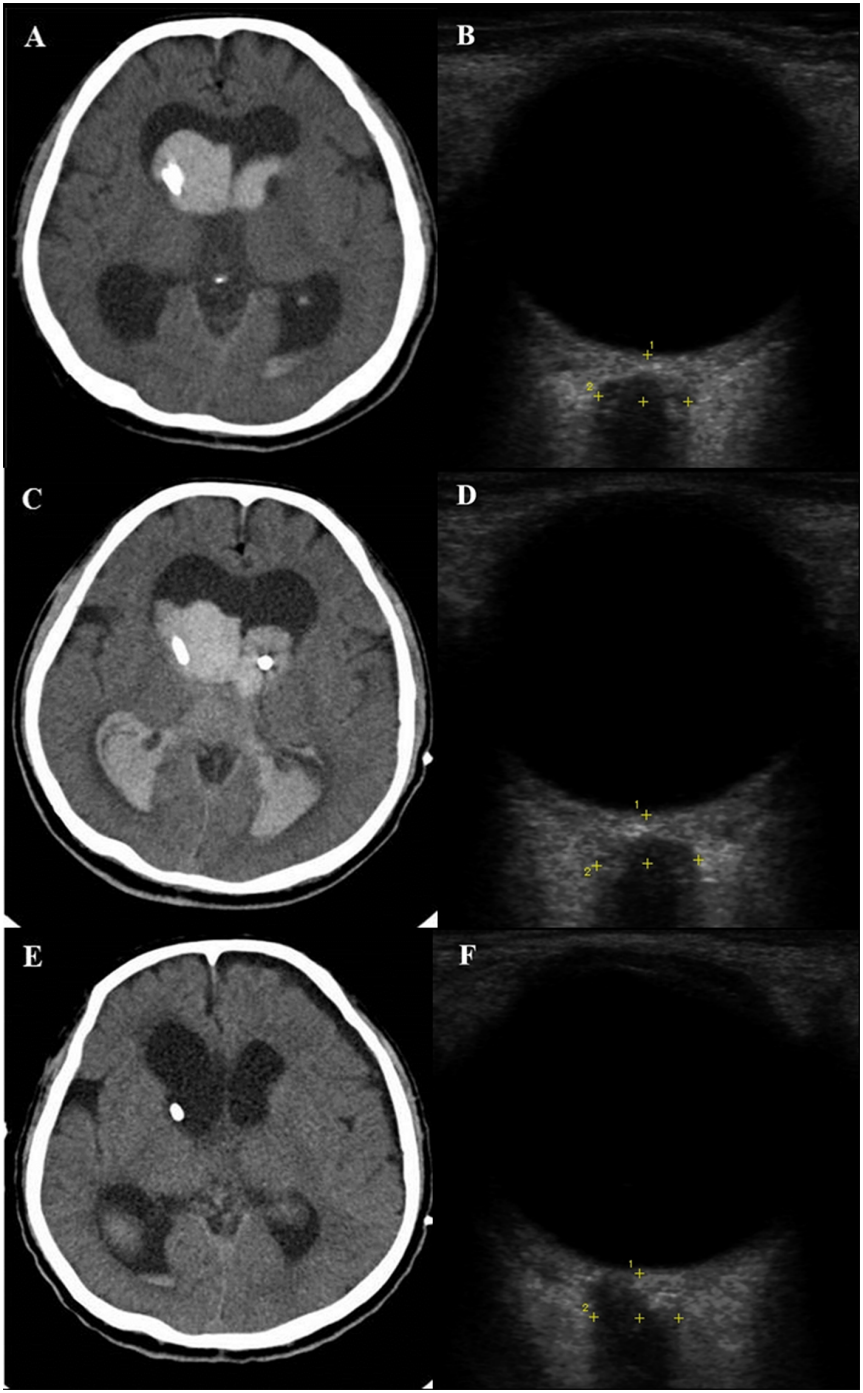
(A, B): A 65-year-old man presented with sudden-onset altered mentality caused by acute IVH and hydrocephalus. US ONSD was measured as 5.7 mm and an EVD was placed in the right lateral ventricle with an opening pressure of 22 mmHg. (C, D): Three hours later, the patient’s ICP surged to 40 mmHg with an ONSD of 6.4 mm due to rebleeding of the right PICA aneurysm. Emergency coil embolization of the right PICA aneurysm was performed and another EVD was inserted in the left lateral ventricle. (E, F): Brain CT scans taken 3 weeks after the operation showed a substantial improvement of hydrocephalus and IVH with an ICP of 10 mmHg and an ONSD of 5.4 mm.
